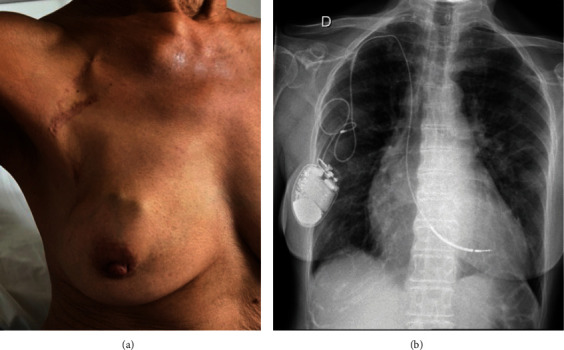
(a) Right thoracic anatomy and (b) anteroposterior chest X-ray.
